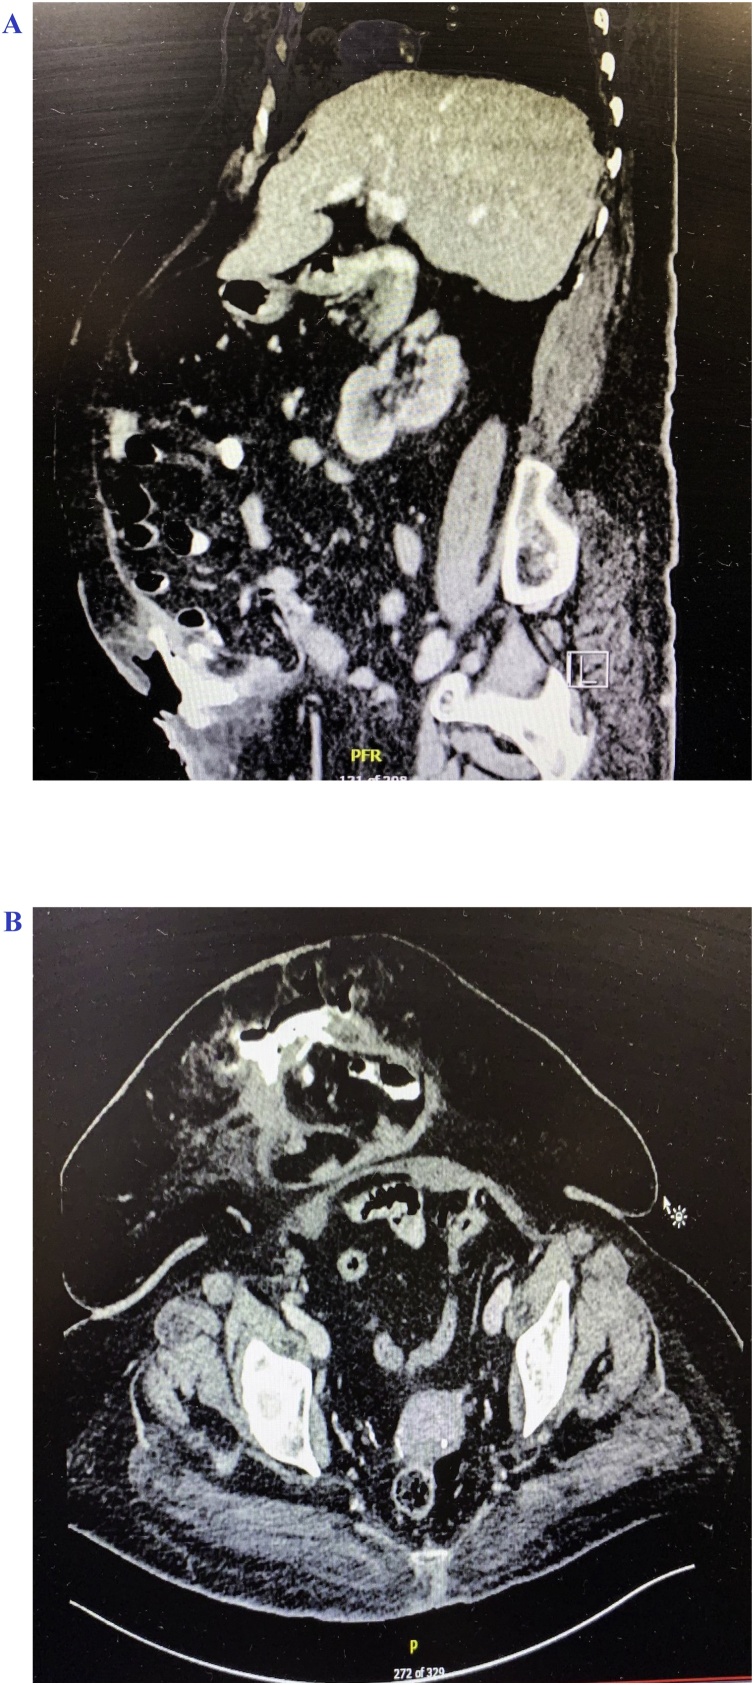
A. CT scan (sagittal images) with PO/IV contrast showing recurrent incisional hernia content with contrast pooling into subcutaneous tissue. B. CT scan (transverse images) with PO/IV contrast further delineating a recurrent incisional hernia with small bowel content and contrast pooling in the subcutaneous tissue.
